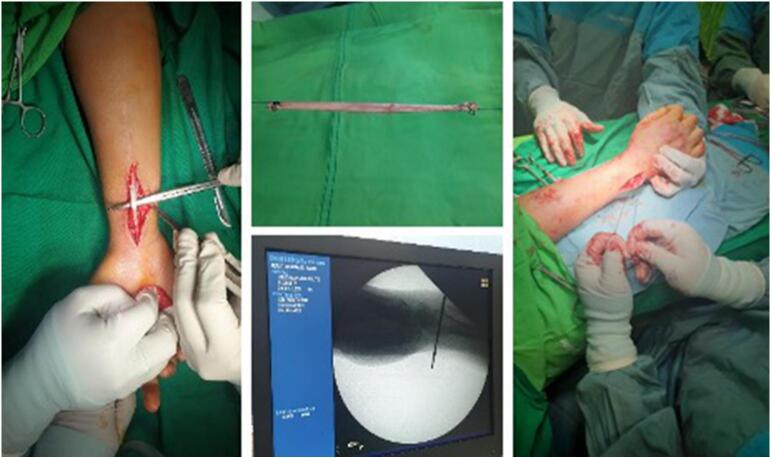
Surgical step of DRUJ reconstruction. Harvesting palmaris longus tendon following ligament reconstruction in chronic instability DRUJ (a). The harvested 10 cm long tendon (b). Lateral X-ray demonstrating guide wire in distal radius (c). The graft was routed on the distal radius from posterior to anterior, then routed to the distal ulna in the fovea (d).
